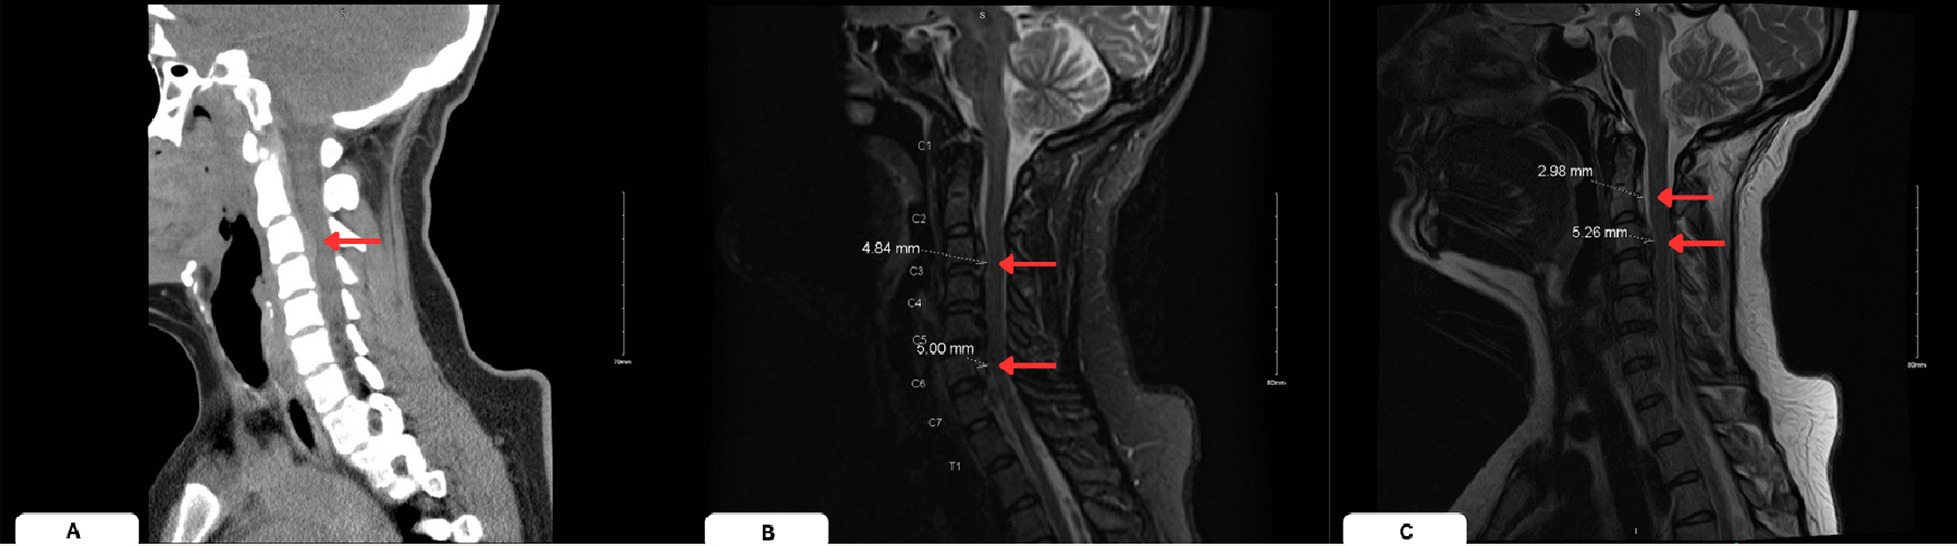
A Sagittal section of computerized tomography cervical spine without contrast displaying anterior cervical epidural hematoma at the level of C3 to C6 (left, red arrow), B Sagittal section of T2 weighted magnetic resonance imaging short tau inversion recovery displaying anterior cervical epidural hematoma at the level of C3 and C6 (middle, red arrows), C Sagittal section of T2 weighted magnetic resonance imaging American college of radiology displaying anterior cervical epidural hematoma
